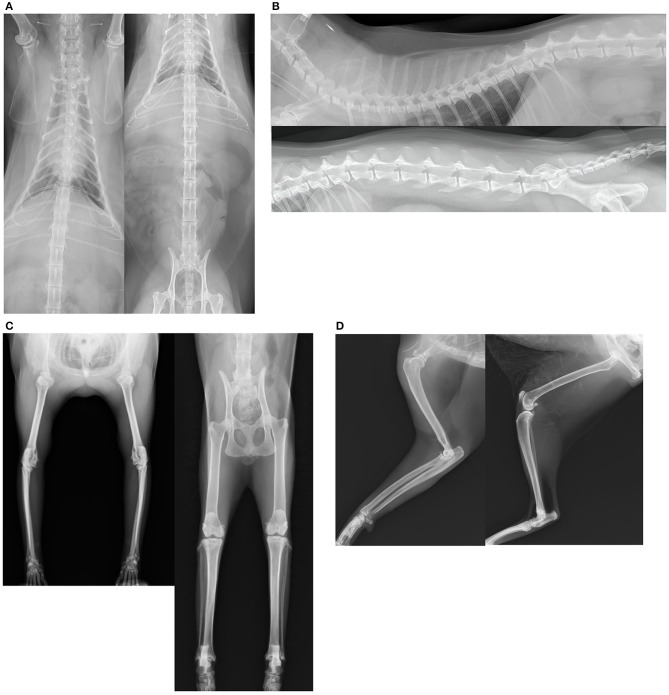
(A–D) Orthogonal radiographs were obtained to examine the intervertebral and appendicular joints.
